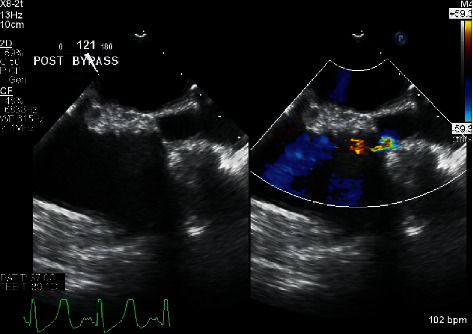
The image in the left panel shows the initial postbypass two-dimensional presurgical midesophageal bicaval TEE view demonstrating superior vena cava stenosis. The image in the right panel is the same with the addition of color flow Doppler demonstrating flow acceleration across the stenosis.
